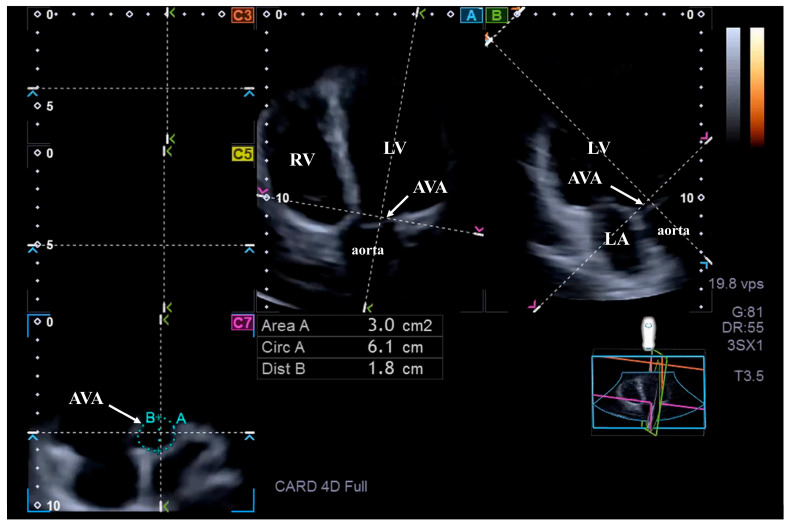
Measurement of the aortic valve annular dimensions by three-dimensional speckle-tracking echocardiography. Following optimization of the LV longitudinal planes on apical 4-chamber view (A) and 2-chamber view (B), and visualization of the aortic valve/aorta by tilting and optimizing the longitudinal planes in these long-axis views (on (A,B)). The planes were positioned to be parallel with the aortic root centerline. The C7 served as the cross-sectional view of the aortic valve annulus, which was perpendicular to the longitudinal plane. The white arrow represents the aortic valve annulus. Abbreviations: AVA = aortic valve annulus; Area = AVA area; Circ = AVA perimeter; Dist B = AVA diameter; LV, left ventricle; RV, right ventricle; LA, left atrium.
